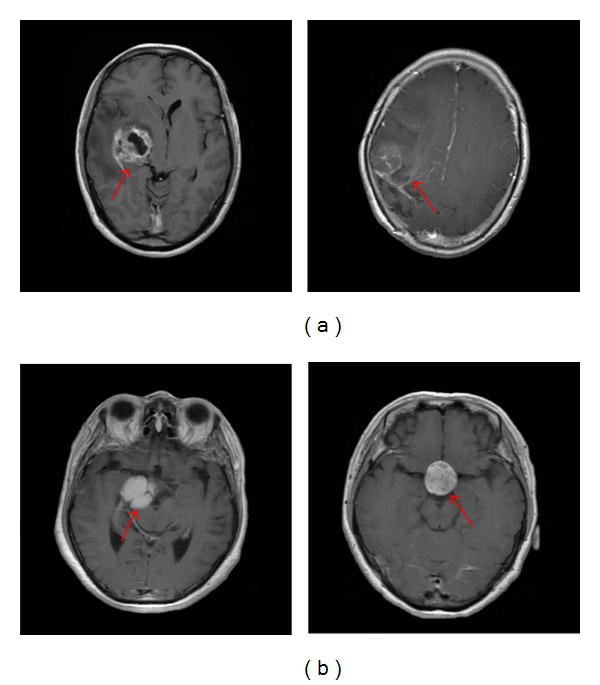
Two examples of brain tumors with varying appearances in T1-weighted CE-MRI images. The red arrows in each image are used to indicate the tumors. (a) Two gliomas in different patients have dissimilar appearances. (b) A meningioma (left) and a pituitary tumor (right) in different patients have similar appearances.
